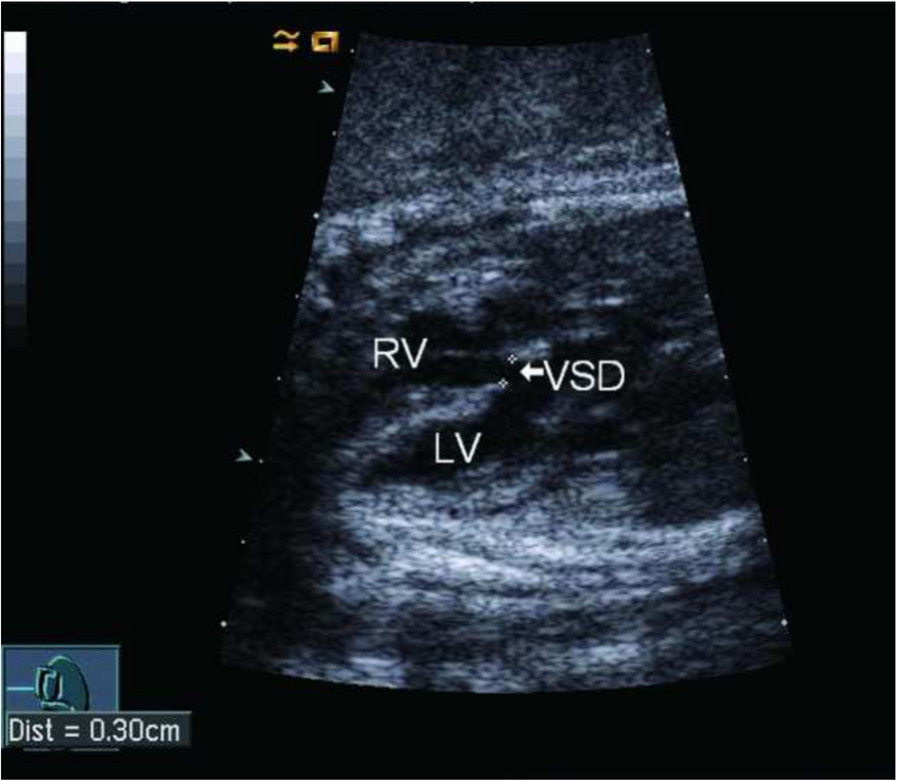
White arrow* indicates a large VSD detected by fetal echocardiography, and no SC during follow-up
